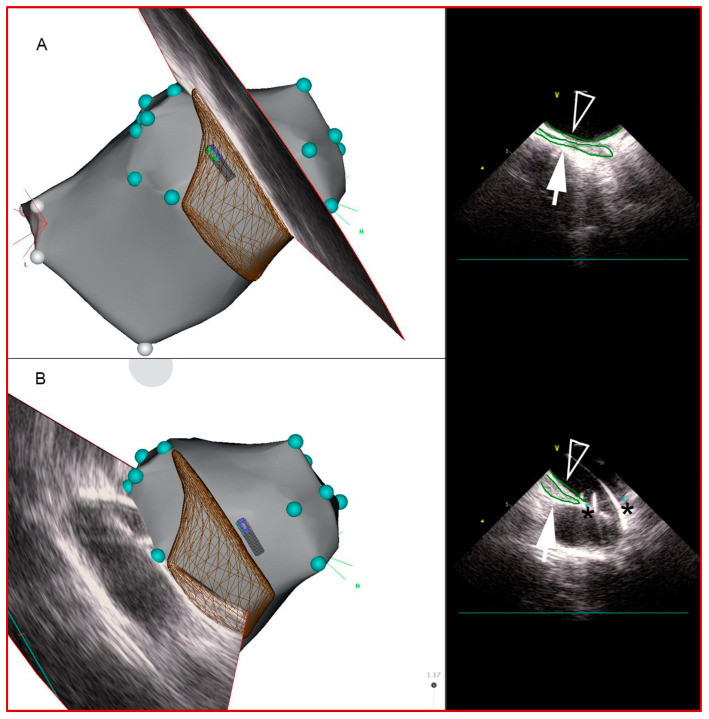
Sequential intracardiac echocardiography (ICE)-derived 2D images of esophagus on CARTO. Esophageal borders (green contours) were traced to create its 3-dimensional course over the LA posterior wall. Panels (A,B). Medial (rightward) and lateral (leftward) esophagus borders, respectively. In panel (B), ICE view, the guidewire is seen going into left inferior PV. Closed arrows: esophageal contours; open arrows: LA posterior wall contours; asterisks: PV ostia.
